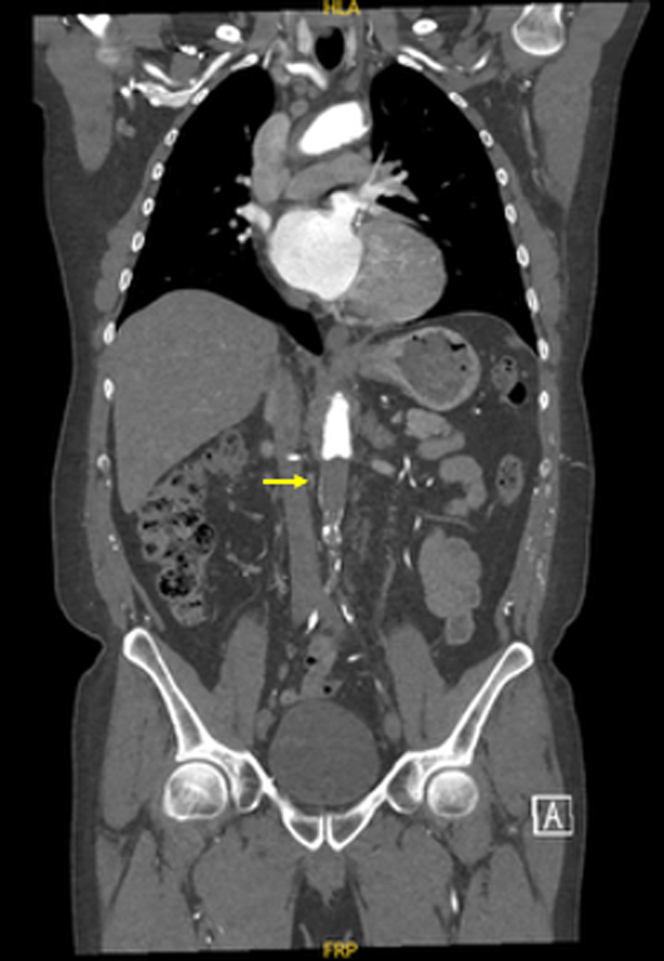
Coronal Image of Computed Tomography Aorta With Arterial Contrast Demonstrating Total Aortic Occlusion
